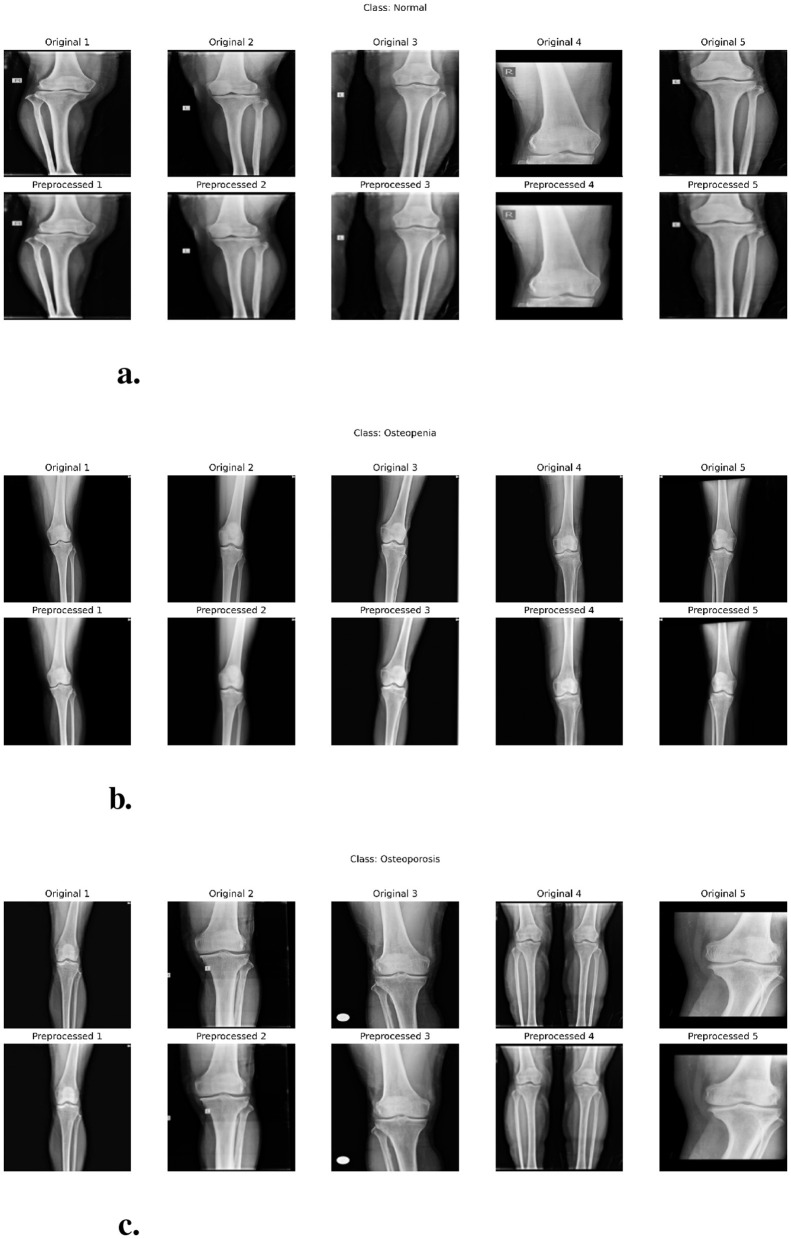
Preprocessing of X-ray images across different conditions. (a) Preprocessing using normal X-ray images using Multi-Class Knee Osteoporosis X-ray Dataset. (b) Preprocessing using Osteopenia X-ray images using Multi-Class Knee Osteoporosis X-ray Dataset. (c) Preprocessing using Osteoporosis X-ray images using Multi-Class Knee Osteoporosis X-ray Dataset.
